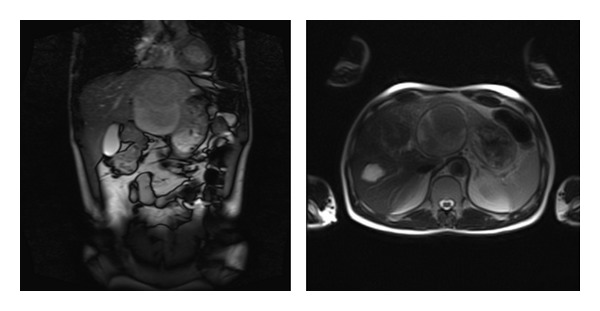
Magnetic resonance imaging sections.
